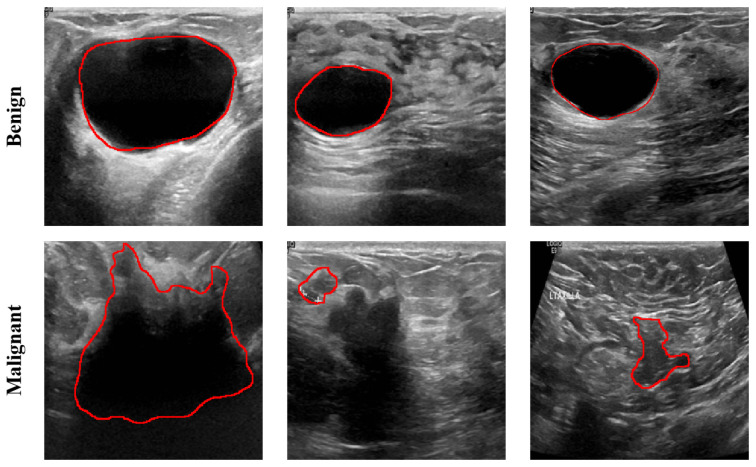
Breast ultrasound benign vs. malignant comparison image, with the red solid line denoting the boundary of the breast tumor.
